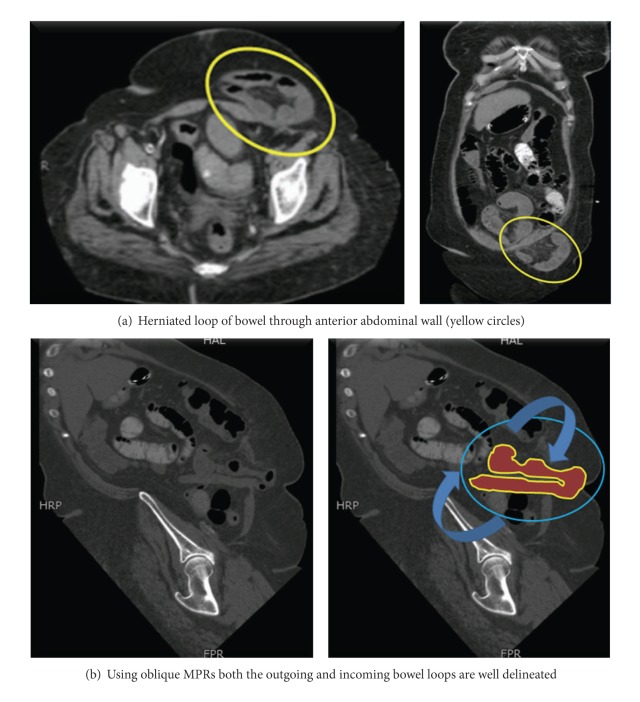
CT scans in a 54-year-old woman with a two-month history of nausea and vomiting acutely presenting with worsening of symptoms. (a) Axial and coronal CT images obtained with intravenous contrast agent show herniated loops of small-bowel loops through a defect in anterior abdominal wall; however, the tract of bowel could not be completely elucidated on axial and coronal sections alone (yellow circles). (b) Oblique reformation exactly shows the outgoing and incoming loops of bowel with proximal dilated segments of bowel representing partial obstruction (blue circles and curved arrows).
